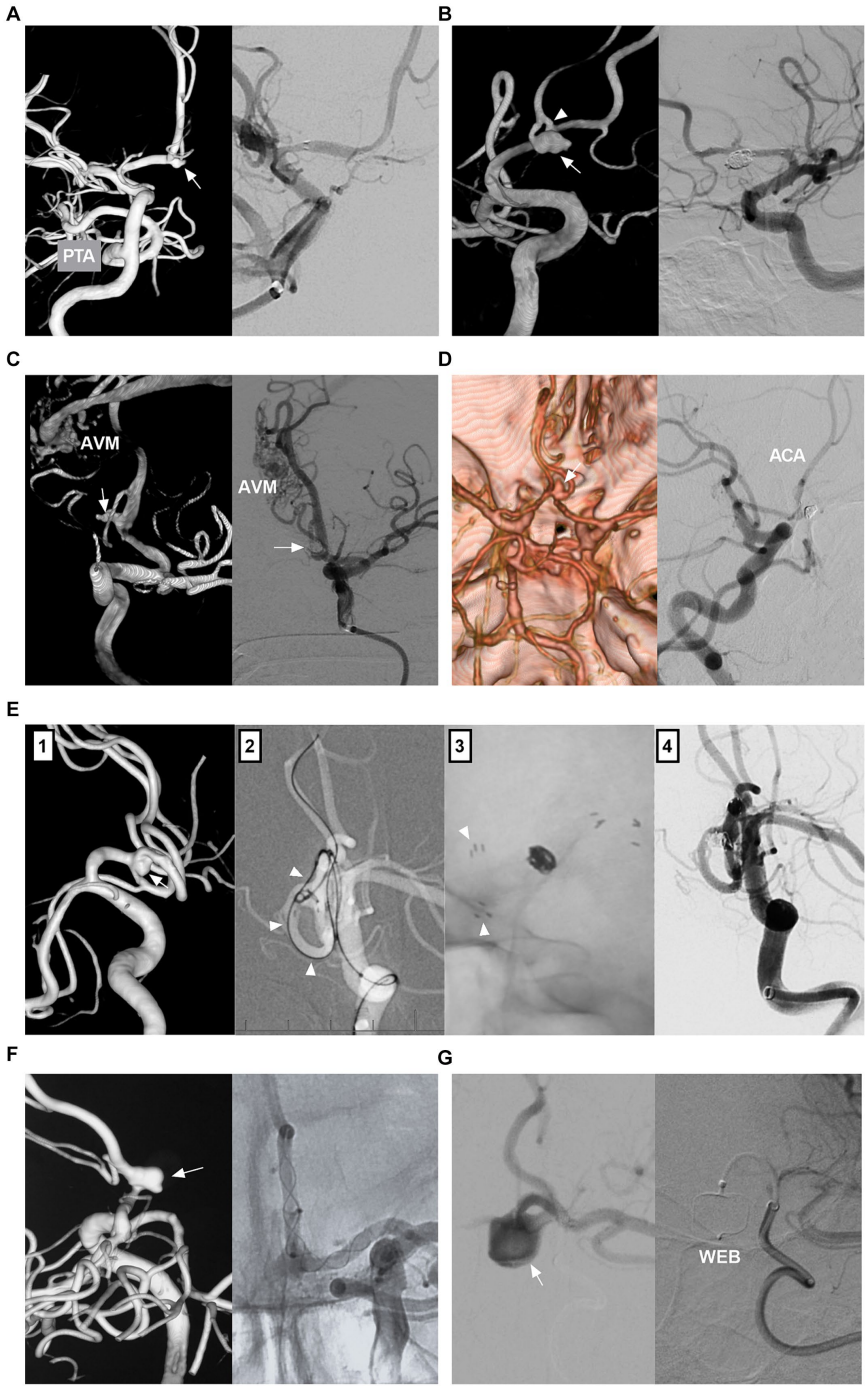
Treatment of AcomA aneurysms. (A) Left panel: 3D-DSA showing an AcomA aneurysm (arrow) with PTA; Right panel: DSA showing the coiled aneurysm with stent assistance. (B) Left panel: 3D-DSA showing an AcomA aneurysm (arrow) in a fenestration (arrowhead). Right panel: Six-month follow-up DSA showing that the aneurysm was occluded completely. (C) Left panel: 3D-DSA showing an AcomA aneurysm (arrow) and an AVM; Right panel: DSA showing the coiled aneurysm with the AVM intact. (D) Left panel: CTA showing an AcomA aneurysm (arrow) with symmetric ACAs; Right panel: Six-month follow-up DSA showing that the aneurysm was coiled completely with AcomA occlusion. (E) Panel 1: 3D-DSA showing an AcomA aneurysm (arrow); Panel 2: Roadmap image showing a microcatheter (arrowheads) crossing the stent into the contralateral ACA to wait to deploy another stent; Panel 3: X-ray image showing the “Y” configure stents, the arrowheads indicate the distal markers of the stent; Panel 4: Postoperative immediate DSA showing the aneurysm was coiled. (F) Left panel: 3D-DSA showing a sidewall AcomA aneurysm (arrow); Right panel: Unsubtracted DSA showing that the aneurysm was covered by FD deployment. (G) Left panel: DSA showing that the AcomA aneurysm with the WEB device in it; Right panel: Unsubtracted DSA showing the WEB device outline. 3D, three-dimensional; A1, first segment; ACA, anterior cerebral artery; AcomA, anterior communicating artery; AVM, arteriovenous malformation; CTA, computed tomography angiography; DSA, digital subtracted angiography; FD, flow diverter; PTA, primitive trigeminal artery; WEB, Woven EndoBridge.
